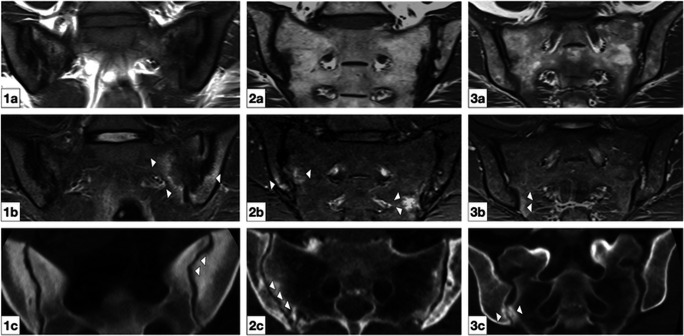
Examples of joint lesions in atypical joints. Row a: T1 weighted oblique coronal MR images. Row b: short-tau inversion recovery (STIR) oblique coronal MR images. Row c: oblique coronal CT reconstructions. Patient 1 (column 1): female patient with osteitis condensans: note the marked sclerosis, the ilium notch, or iliosacral complex (panel 1c, arrowheads) as well as the bone marrow edema on STIR imaging (panel 1b, arrowheads). Patient 2 (column 2): female with osteoarthritis of the SIJ: note the overall concave shape of the ilium (panel 2c, arrowheads) and the marked bone marrow edema adjacent to the joint on STIR imaging (panel 2b, white arrowheads). Patient 3: female with osteitis condensans. An accessory joint is seen almost exclusively on CT (panel 3c, arrowheads), but a faint bone marrow edema can be seen in STIR imaging (panel 3b, arrowhead)
